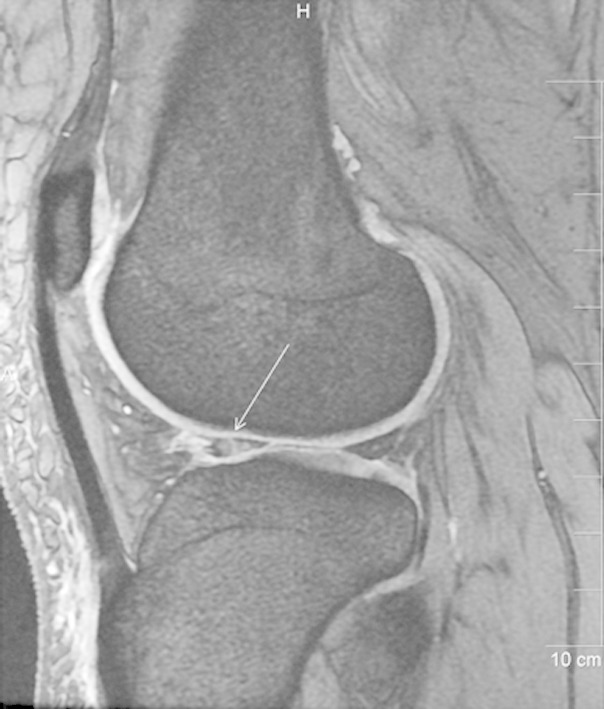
Sagittal T2-weighted MR image reveals tear in the anterior horn of lateral meniscus that appears globular signal of increased intensity extending to superior and inferior articular surfaces. (arrow) The tear was not recognized at arthroscopy performed 7 days after MR imaging and therefore constituting a false-positive diagnosis at MR.
